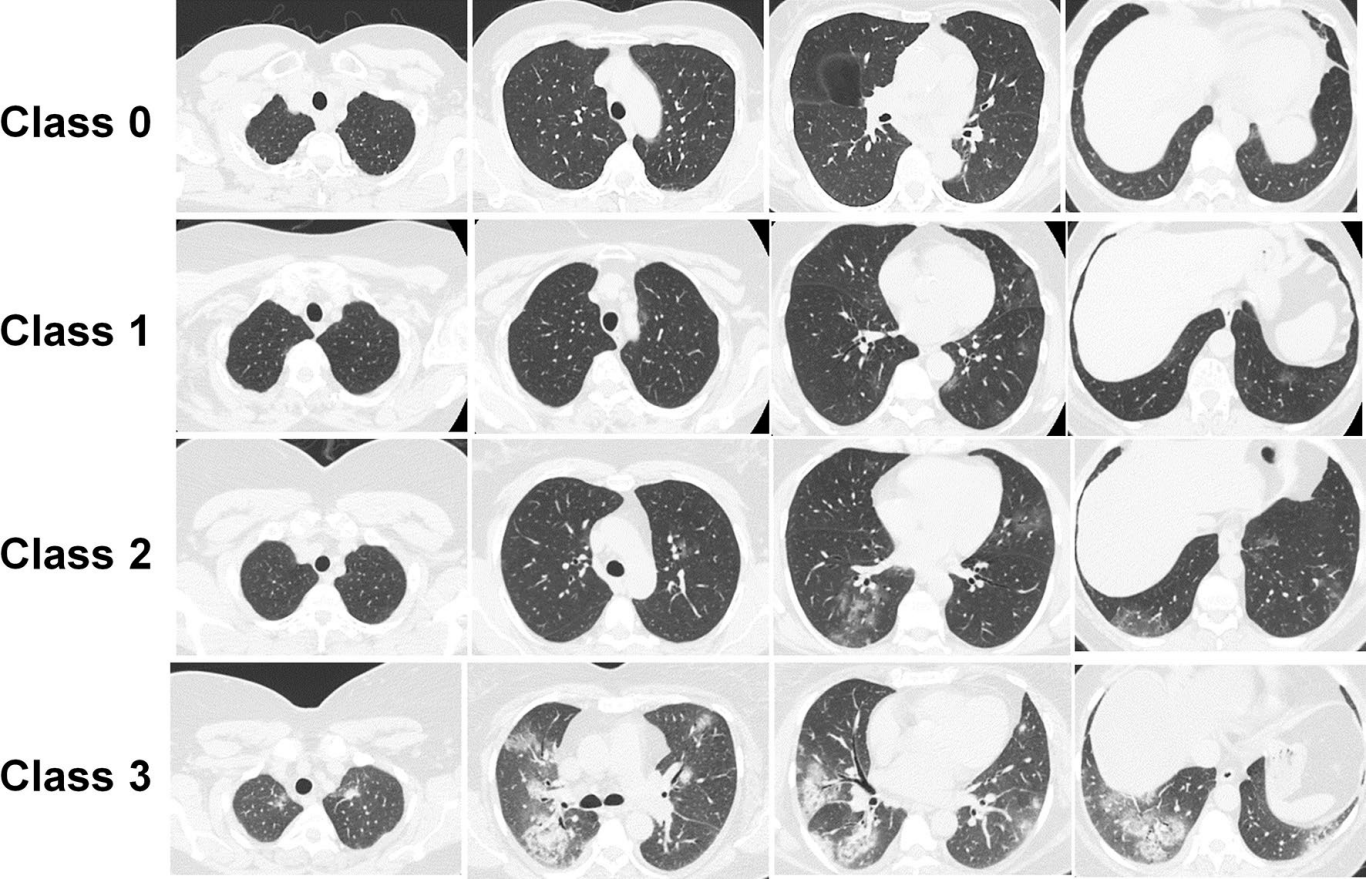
Examples of patient CT images belonging to different classes with different scores.
